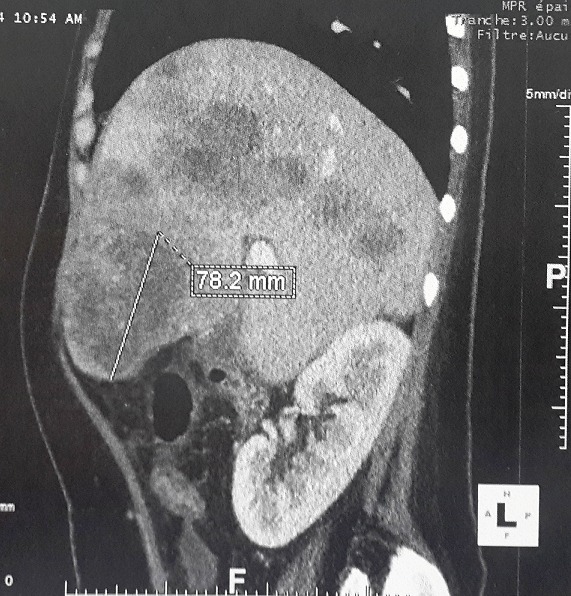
Abdominal CT scan demonstrating multiple enhancing hypodense lesions of the liver
